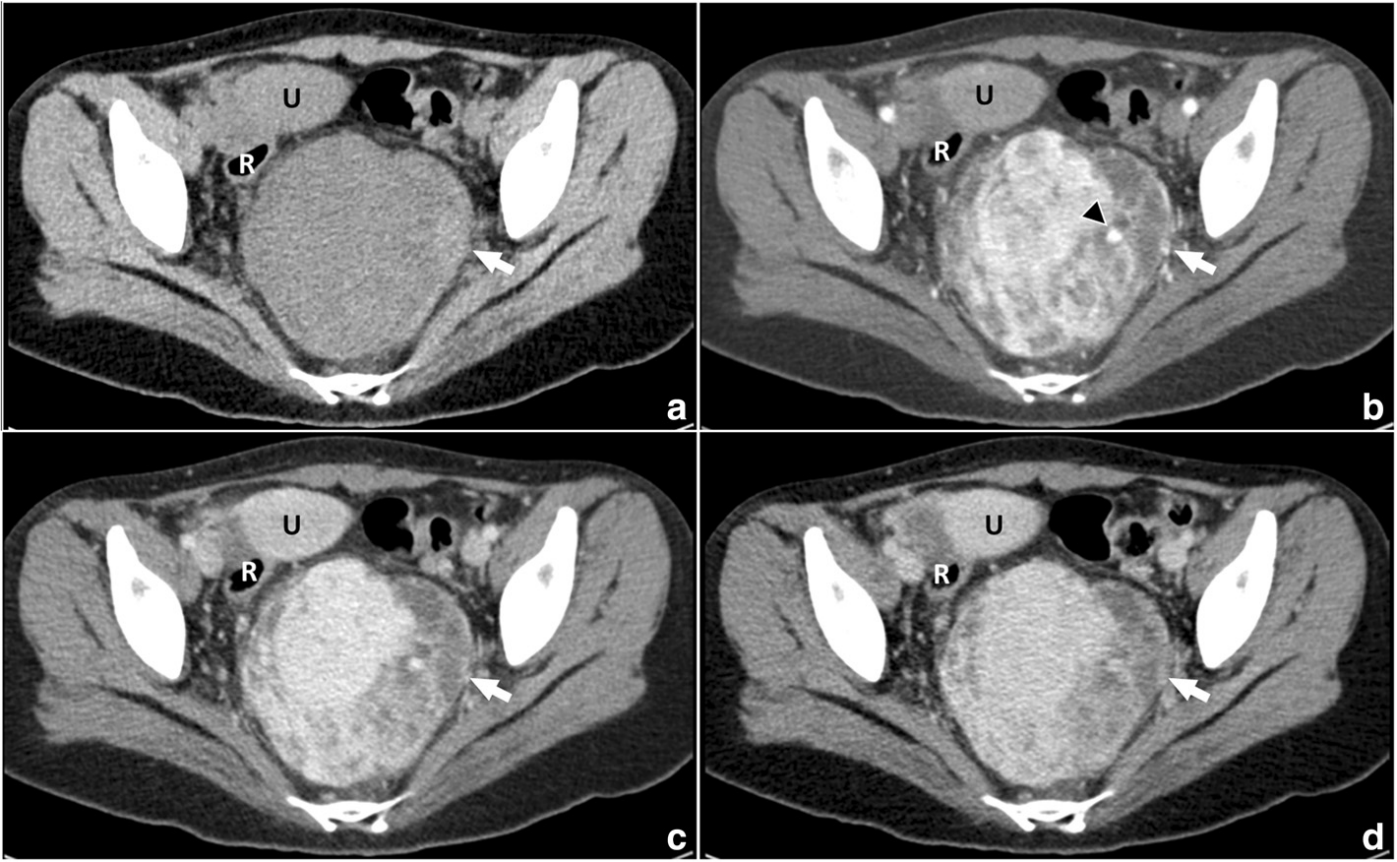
CT examination of the pelvic cavity. Plain CT scan (a) shows a well-defined mass with slightly inhomogenous density in the pelvic retroperitoneum. The left part of the mass has higher density (arrow). On contrast-enhanced CT images (b), it shows marked but heterogenous enhancement during arterial phase. Dilated feeding artery shows clearly in the mass (arrow). The enhanced area increases but the CT value decreases in the following phases (c-d). Areas with higher density showed on plain CT scan has no obvious enhancement (arrow). Some irregular area without obvious enhancement in the inner part is also detected. U: uterus, R: rectum
